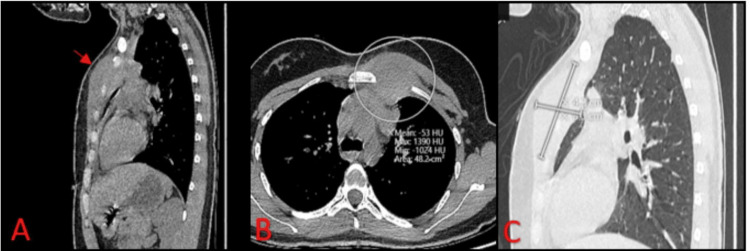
Computed tomography scans of the chest A: the sagittal mediastinal view showed an anterior chest wall mass (red arrow); B: the axial mediastinal view showed an anterior chest wall mass (circle), suggestive of an abscess or liquefied hematoma; C: the sagittal lung view showed the dimensions of the mass to be about 8*4 cm.
